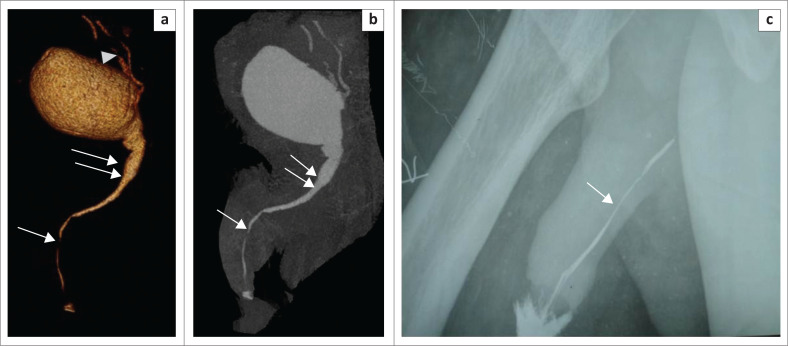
(a) Volume rendering technique image (multidetector computed tomographic urethrography [MDCTU]) showing a long-segment anterior urethral stricture (arrow) with adequate visualisation of the urinary bladder and posterior urethra (double arrows); both lower ureters (arrowheads) are opacified because of intravenous contrast administration. (b) Sagittal maximum intensity projection (MDCTU) showing the long-segment anterior urethral stricture (arrow) with adequate visualisation of the urinary bladder and posterior urethra (double arrows). (c) Retrograde urethrography image of the same patient indicating the long-segment anterior urethral stricture (arrow), with no passage of contrast into posterior urethra. Micturating cystourethrography could not be performed in this patient due to unsuccessful catheterisation.
